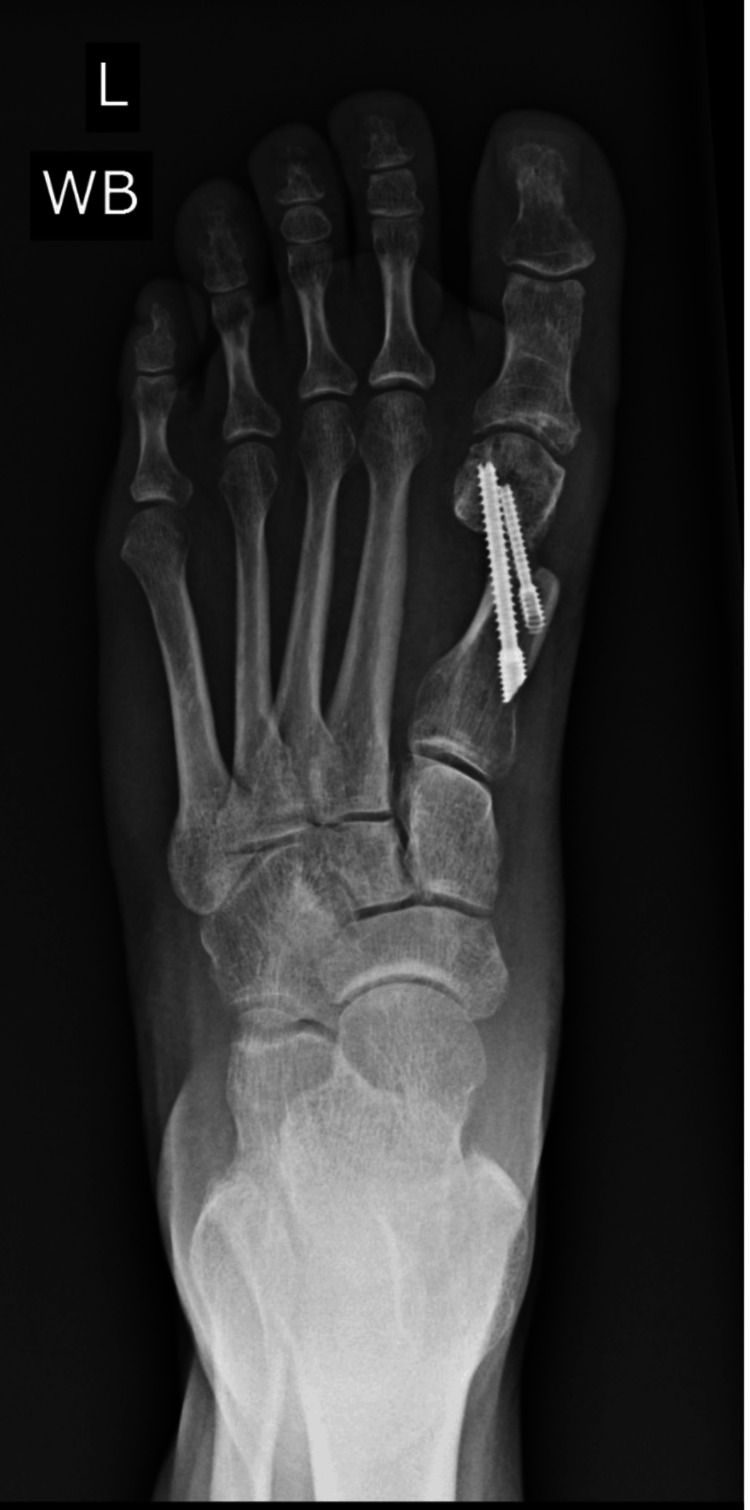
Radiograph five months postoperatively demonstrating persistent radiolucency at the osteotomy site, consistent with nonunion
